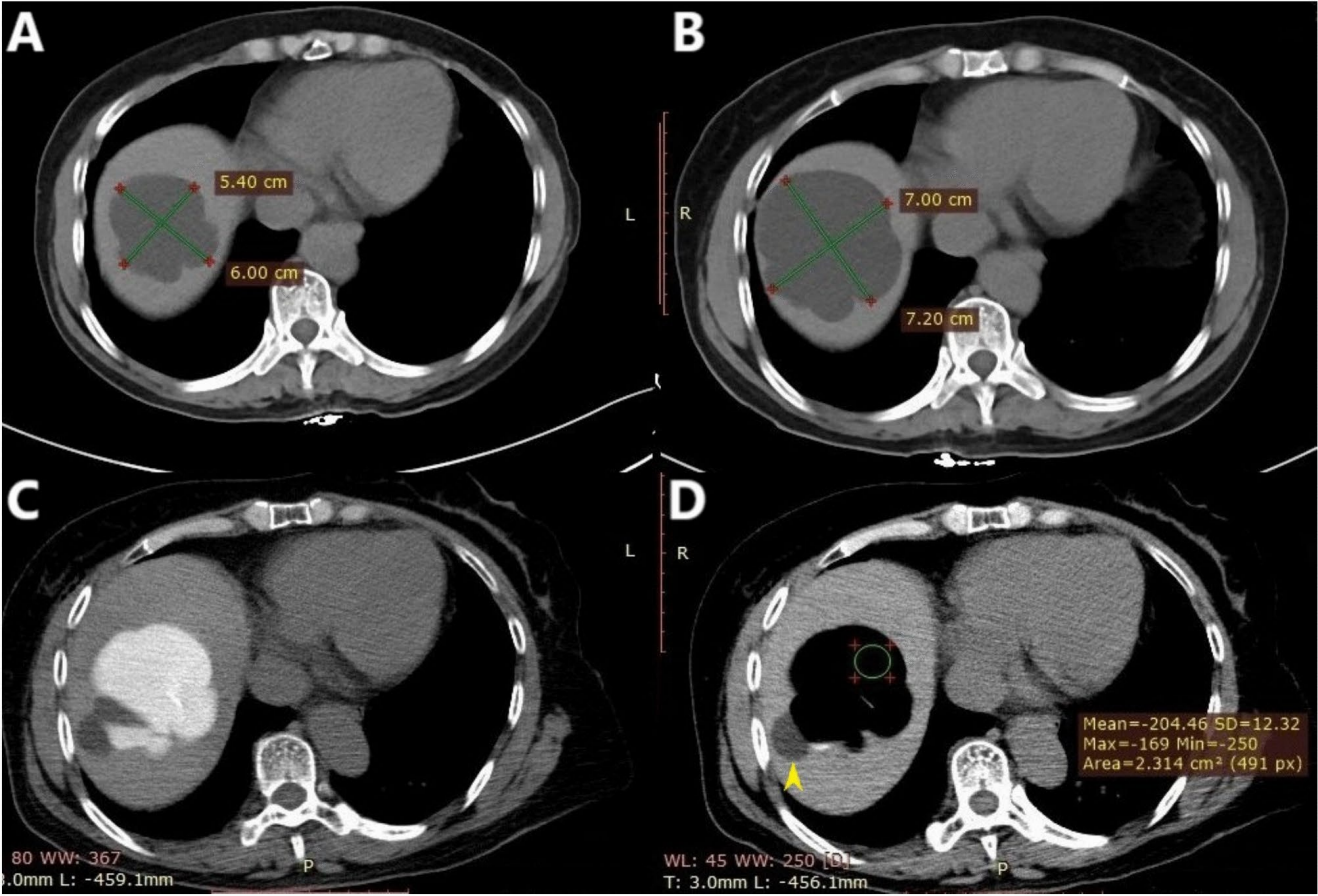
A 57-year-old woman presented with an enlarging hepatic cyst. The simple hepatic cyst (approximately 3–4 cm in size) was accidentally discovered in 2016 via ultrasound. CT performed in May 2018 and November 2019 revealed progressive enlargement of the cyst. The procedure was performed at Center A using the modified technique, and the patient’s prior (A, B) and interprocedural (C, D) CT images are shown below. (A) In May 2018, the cyst size was 5.4 cm × 6.0 cm × 3.6 cm. (B) In November 2019, the cyst size was 7.0 cm × 7.2 cm × 4.6 cm. Its volume increased from approximately 60 mL in May 2018 to 121 mL in November 2019. (C) In December 2019, the patient underwent percutaneous drainage. The needle tip was inserted close to the deep cyst wall, after which the contrast agent was injected. (D) The CT value of the intracystic fluid was − 204 HU, suggesting that the ethanol concentration exceeded 90%. A small cyst (yellow arrowhead) adjoining the large cyst was not treated.
